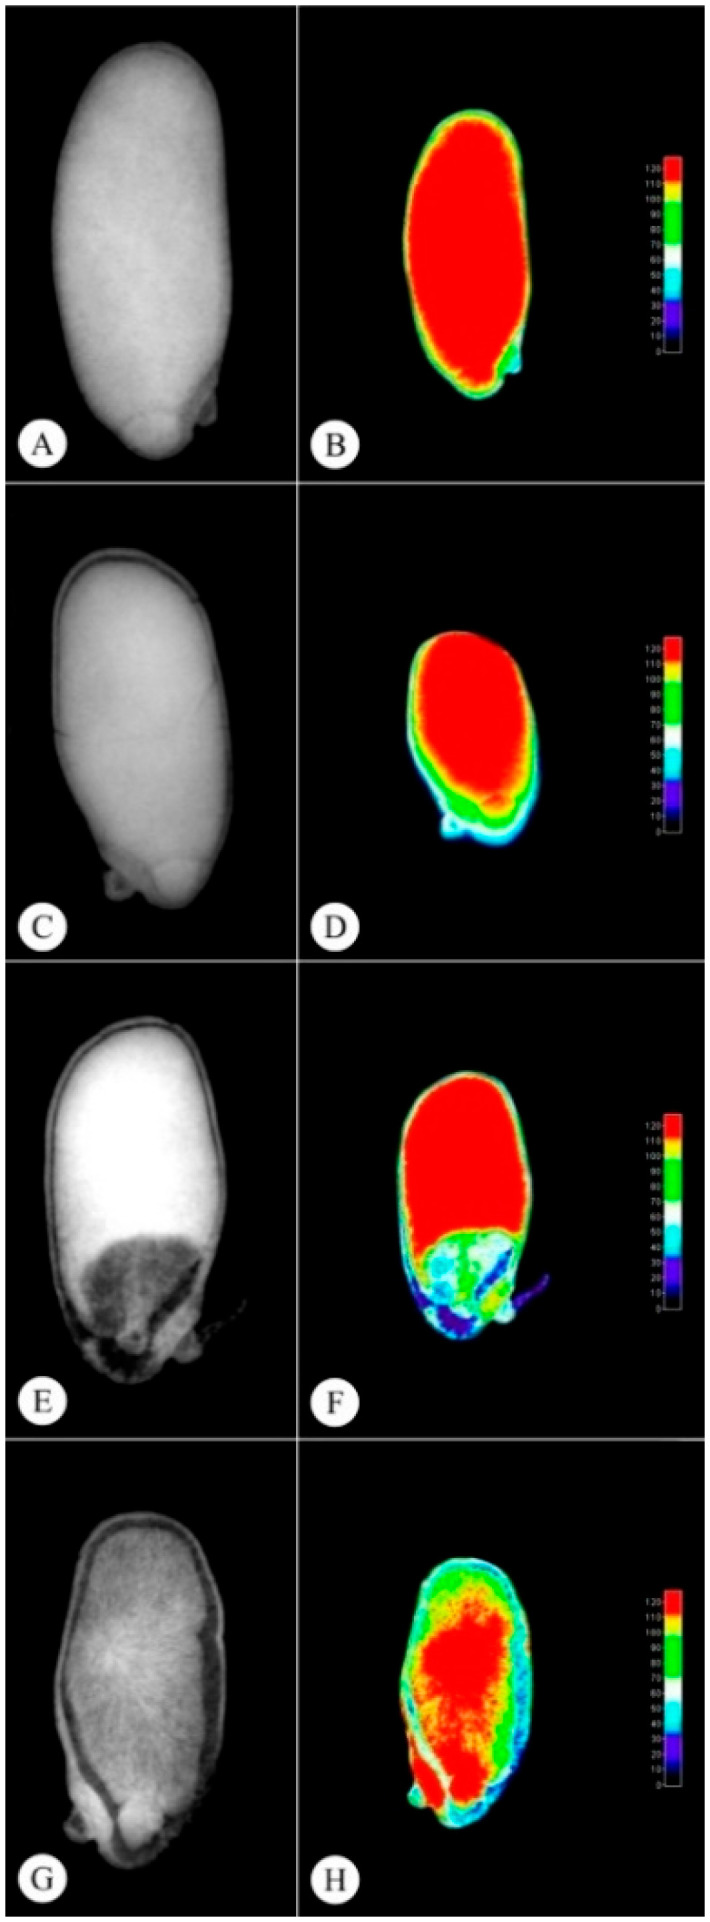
Pterodon pubescens* radiographic seed images and 3D (A,B) yellow seeds, (C,D) light brown seeds, (E,F) dark brown seeds and (G,H) black seeds.
